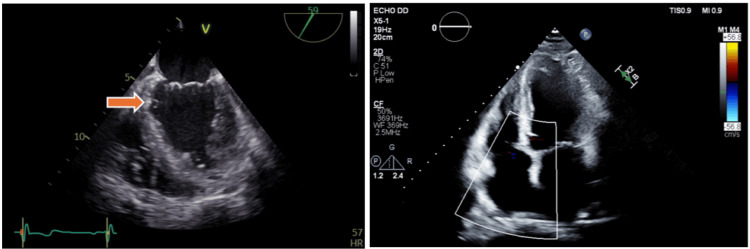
TTE of the Left Ventricle A 12 × 4 mm filamentous mass attached to the interventricular septum just below the mitral valve (orange arrow), suggestive of a mobile intracardiac mass, visualized in both parasternal short-axis and apical four-chamber views. TTE=transthoracic echocardiograph
